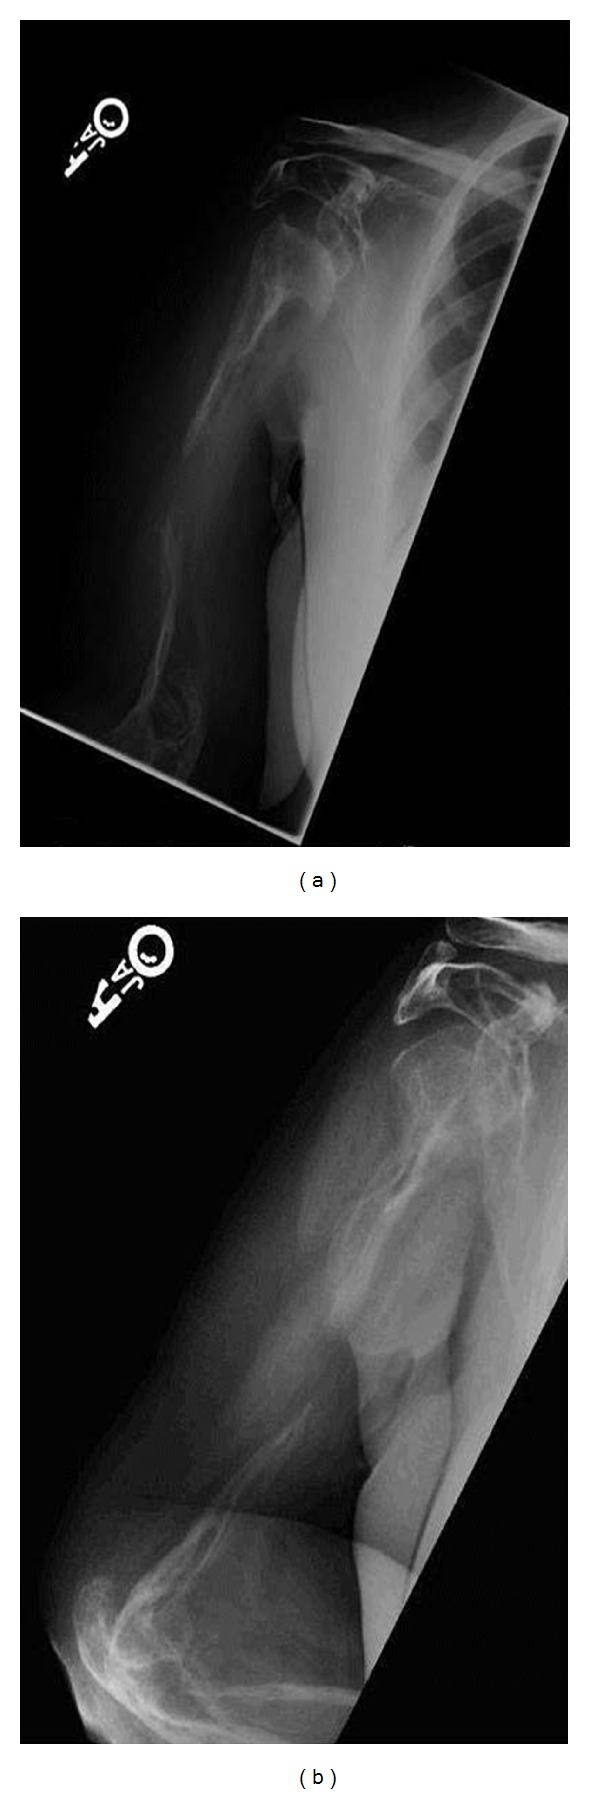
Anteroposterior and lateral plain radiographs of the humerus with diffuse osteopenia and extensive radiolucent foci in the intramedullary and subcortical regions of the bone, and disappearance of the midshaft humerus with tapering of the bone ends. Multiple lytic lesions of the scapula are also visible.
